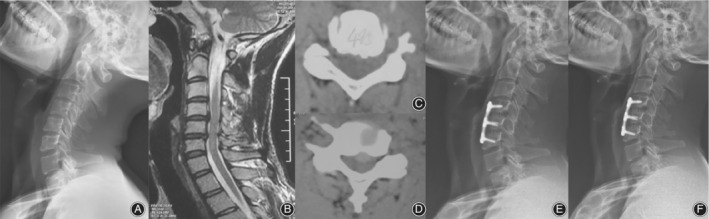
A 41‐year‐old male patient with proximal‐type CSA at the C_4/5_ and C_5/6_ levels. (A) The pre‐operative X‐rays of the cervical spine showing narrowed intervertebral space of the C_4/5_ and C_5/6_ levels. (B) Sagittal T2‐weighted magnetic resonance image showing cord compression at the C_4_‐C_6_ space. (C) CT image at the C_4_‐C_5_ space showing impingement against C_4/5_ intervertebral disc on the left side. (An intraoperative exploration confirmed that multiple nucleus pulposus broke into the spinal canal and the nerve root outlet). (D) CT image at the C_5_‐C_6_ space showing that the central C_5/6_ disc protruded to the left and the outlet of the left nerve root narrowed. (E) postoperative lateral X‐ray of the cervical spine showing that the internal fixation is in good position. (F) Follow‐up at 6 months, X‐ray of the cervical spine showing that the internal fixation is firm and solid arthrodeses is formed.
